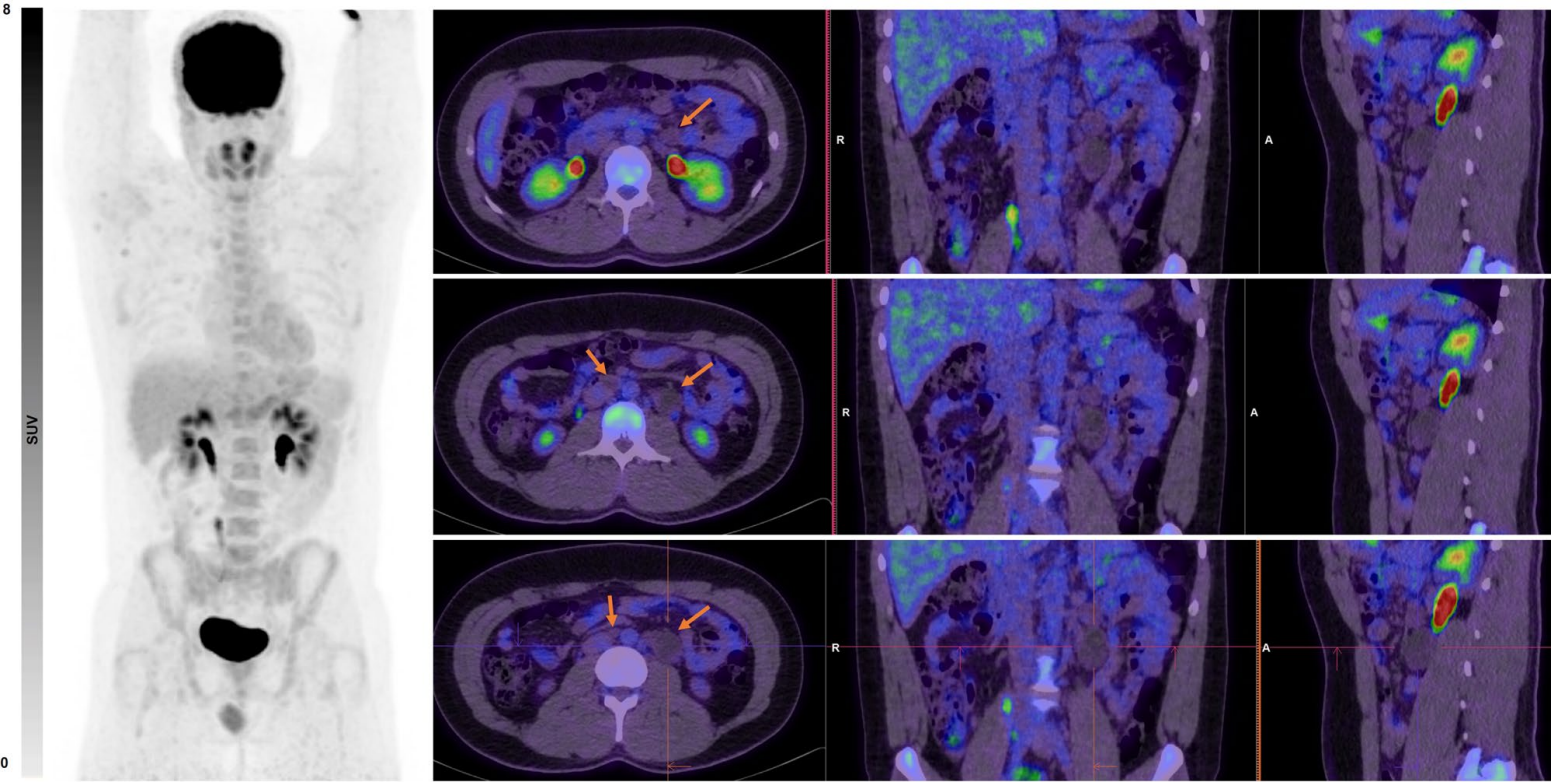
A 30-year-old male with a non-seminoma germ cell tumor, previously treated with a left orchidectomy. The PET scan demonstrates multiple low-density nodal lesions in the retroperitoneum, including at the aortocaval and left para-aortic stations (red arrows), with no increased FDG uptake. The patient subsequently underwent retroperitoneal lymph node dissection, which revealed multiple nodal metastases
